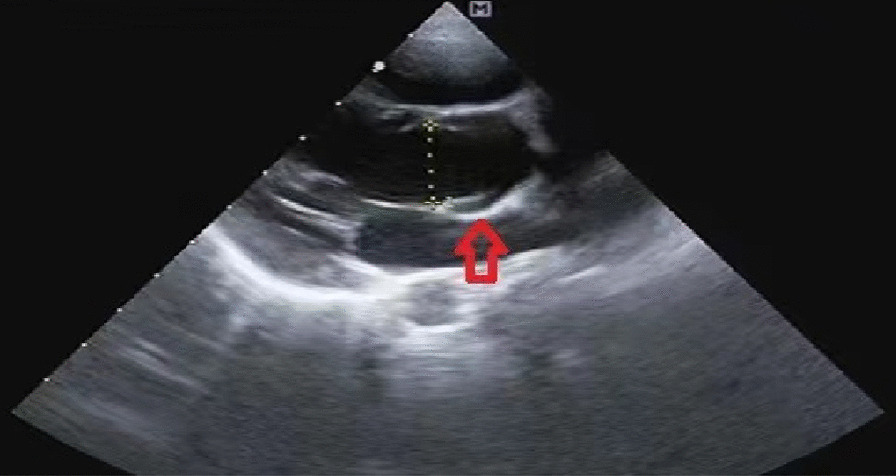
Echocardiographic image of aortic root. *Note*: the red arrow indicates aortic sinus of Valsalva
